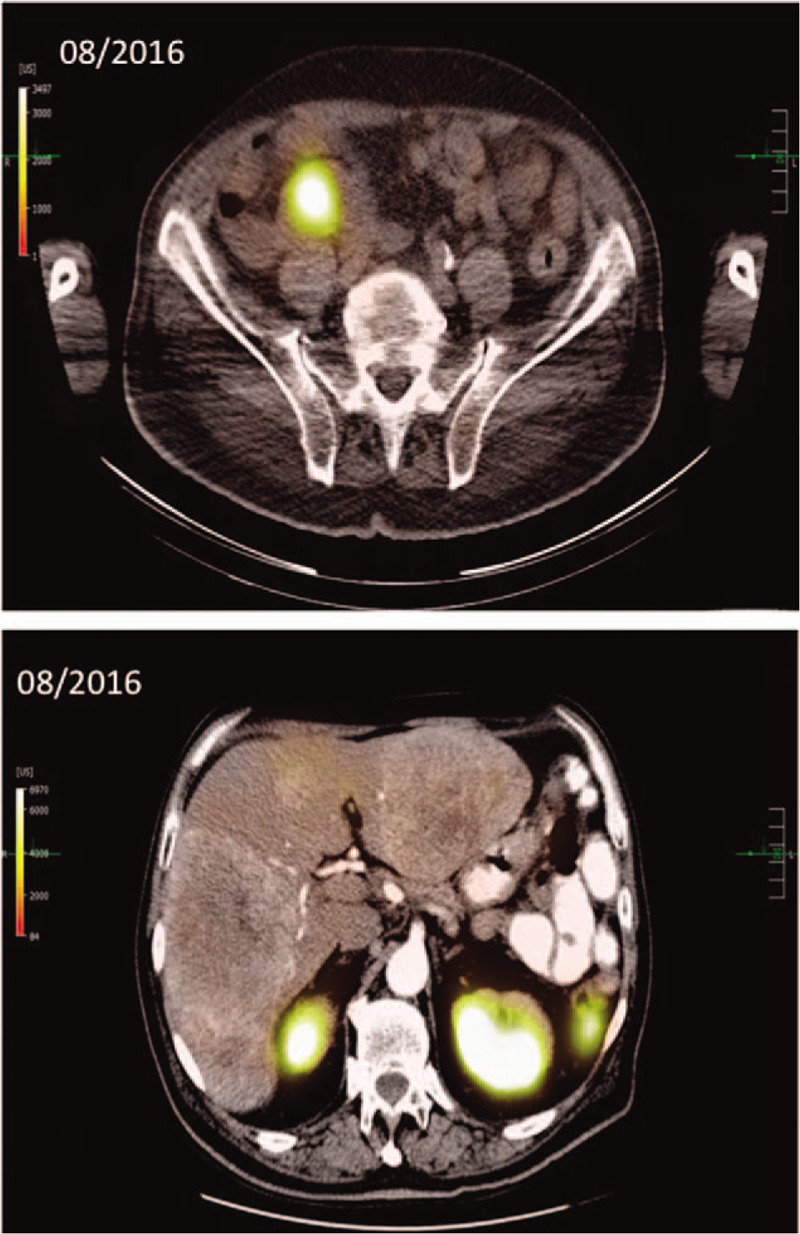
Initial DOTATOC-PET/CT imaging of a functional ileal NET G2 with synchronous hepatic metastases reveals: A) Somatostatin-Receptor (SSR) positive ileal primary tumor and B) several SSR negative hepatic metastases (initial hepatic tumor load 50%). PET/CT = positron emission tomography/computed tomography. SSR = somatostatin receptor.
